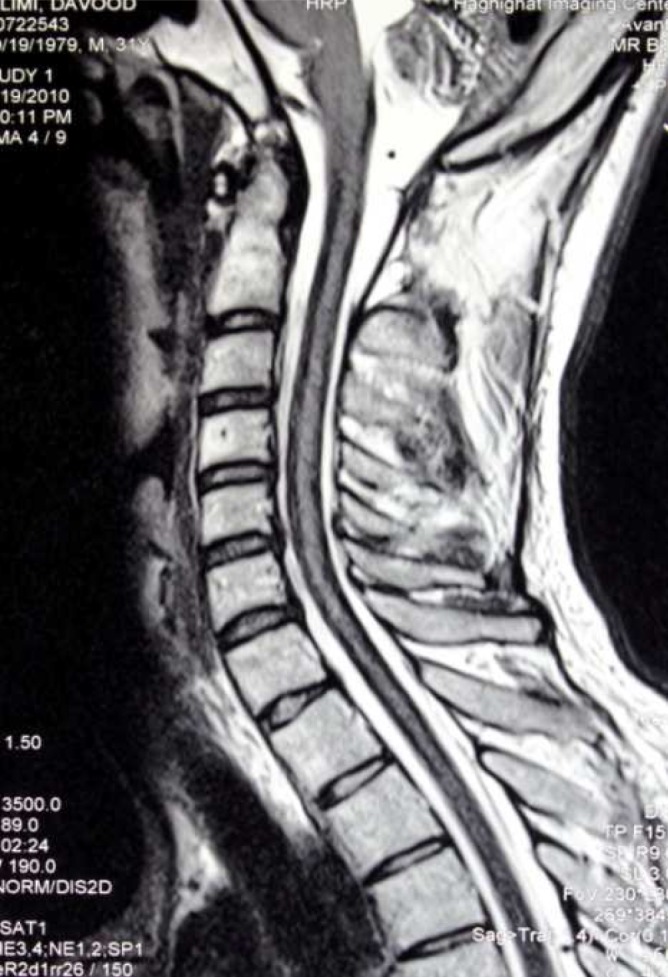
Sagittal T2W1 cervical magnetic resonance imaging (MRI) showed iron deposition in cord as low signal intensity
